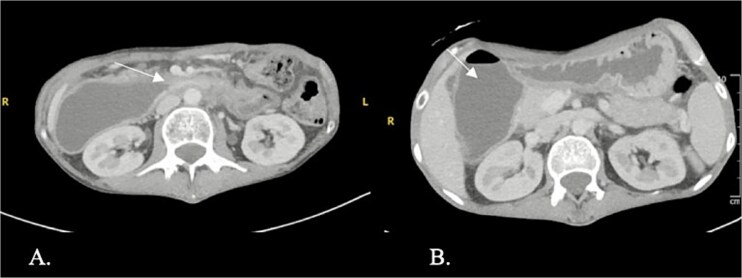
Axial contrast-enhanced computed tomography (CT) showing a stricture with thickening in the third and fourth parts of the duodenum (arrow) and proximal duodenal and gastric dilatation.
